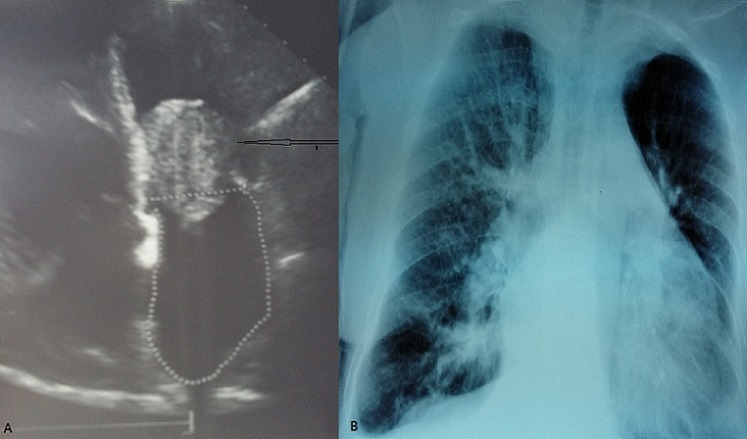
A) échocardiographie coupe 4 cavités montrant un myxome envalé dans la valve mitrale (tête de flèche); B) radiographie pulmonaire de face montrant un infiltrat alvéolo-interstitiel bilatéral faisant évoqué un œdème aiguë du poumon
